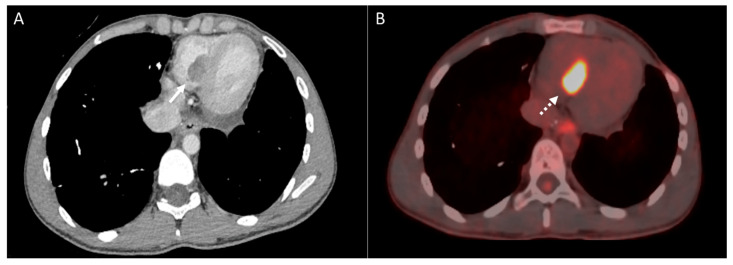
Patient with osteosarcoma and cardiac metastasis. Axial enhanced (A) and axial fused PET-CT (B) images show right ventricular tumor thrombus (arrow), which demonstrates FDG avid uptake (dashed arrow).
